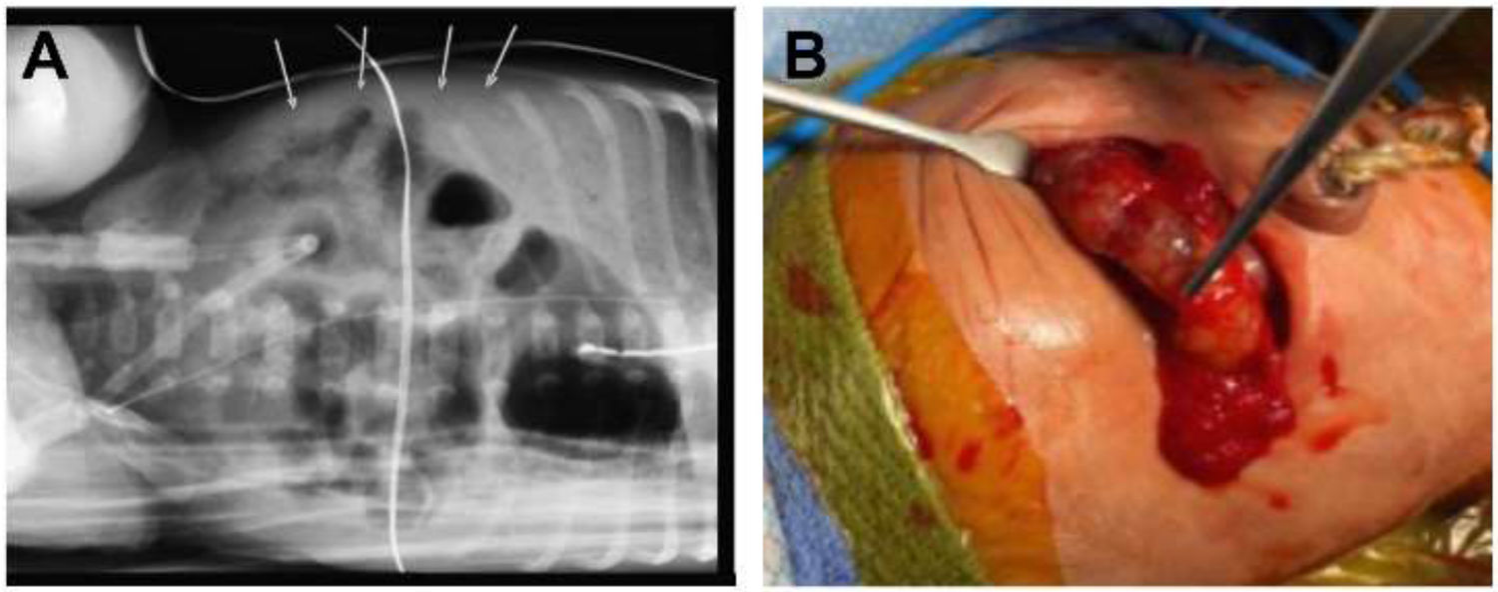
Radiologic and clinical findings in necrotizing enterocolitis. A. Abdominal x-ray revealing the presence of pneumatosis intestinal and intestinal dilation in a child with NEC; B. The intestinal findings in the patient in A, revealing the presence of intestinal ischemia and necrosis.
